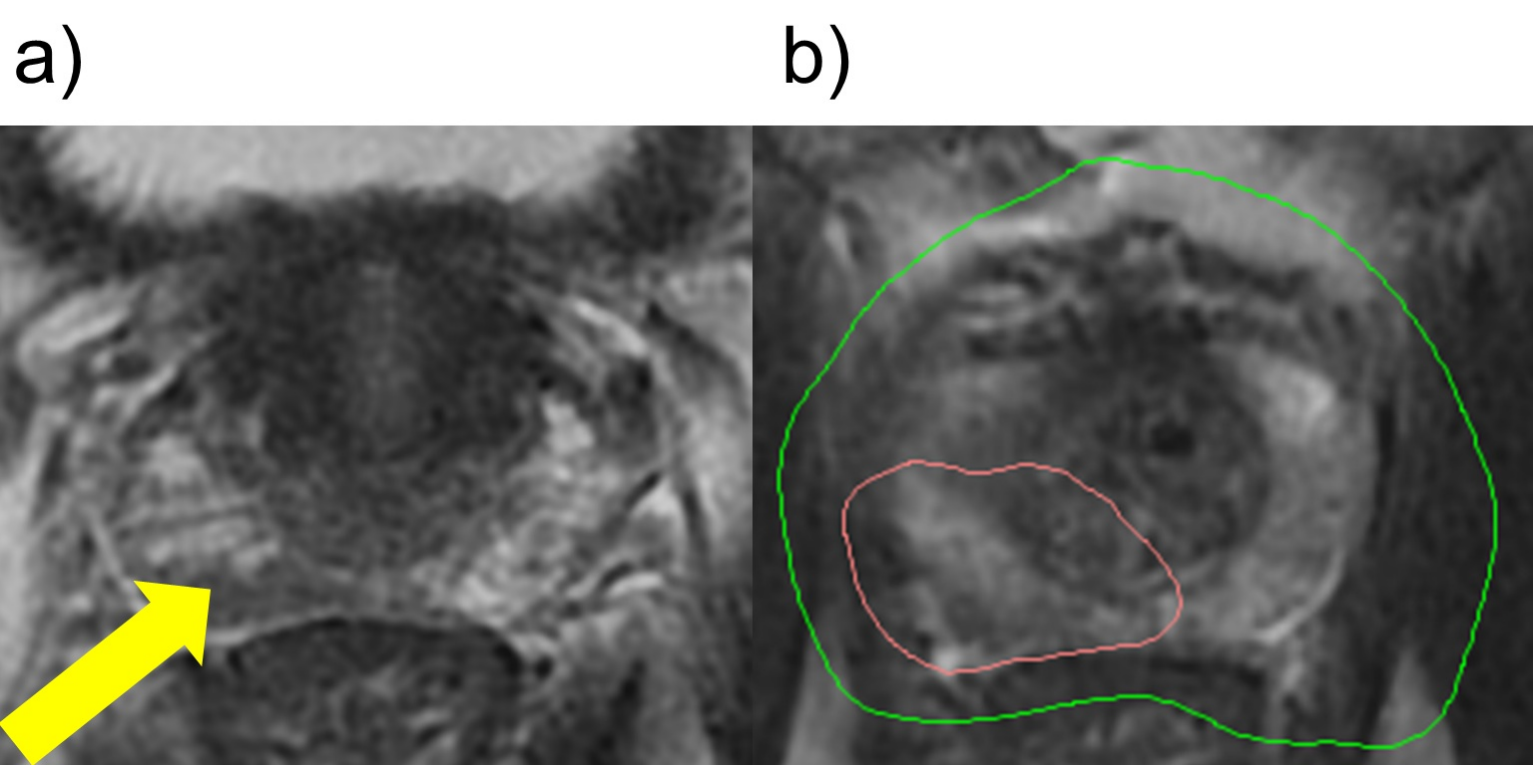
Case 2 shows a right posteriolateral peripheral zone lesion treated with stereotactic body radiotherapy. (a) Hypointense, right posteriolateral peripheral zone lesion with suspicious radiographic features (indicated by arrow). (b) Green and red distributions indicate 36.25 Gy and 40 Gy radiation isodose lines, respectively.
